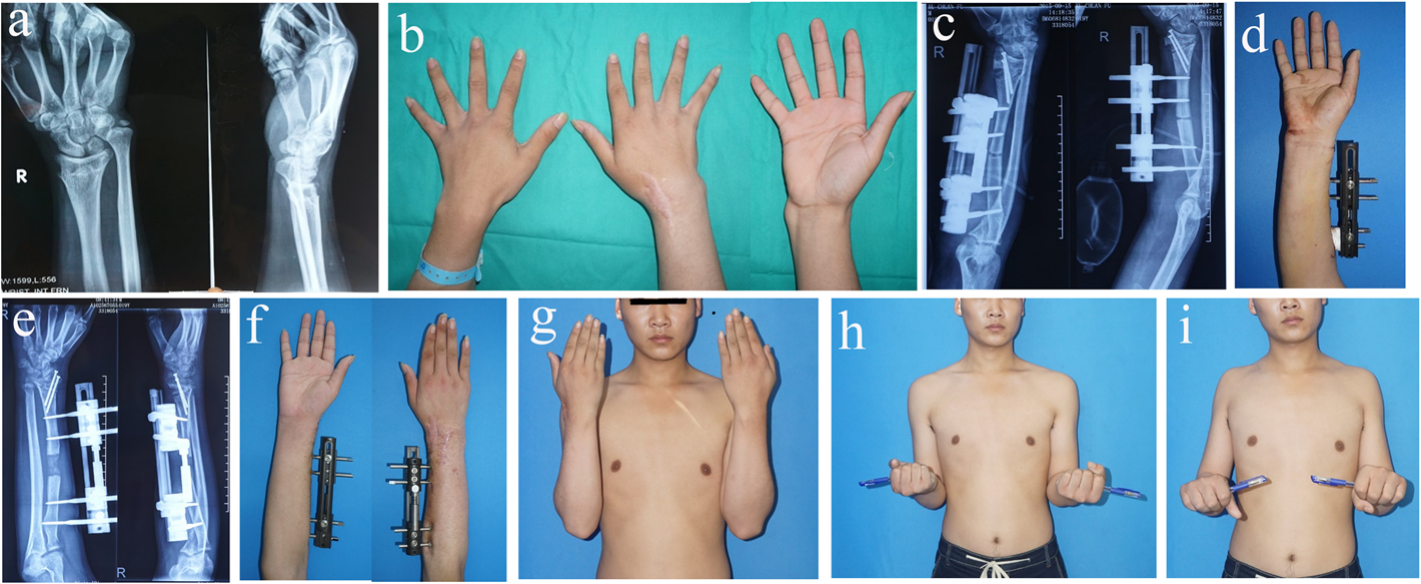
a, b A patient suffered radial shortening with distal ulnar-radial dislocation. c, d Postoperative X-ray. e, f X-ray and gross observation when distraction was terminated. g, h The cosmetic and functional results
